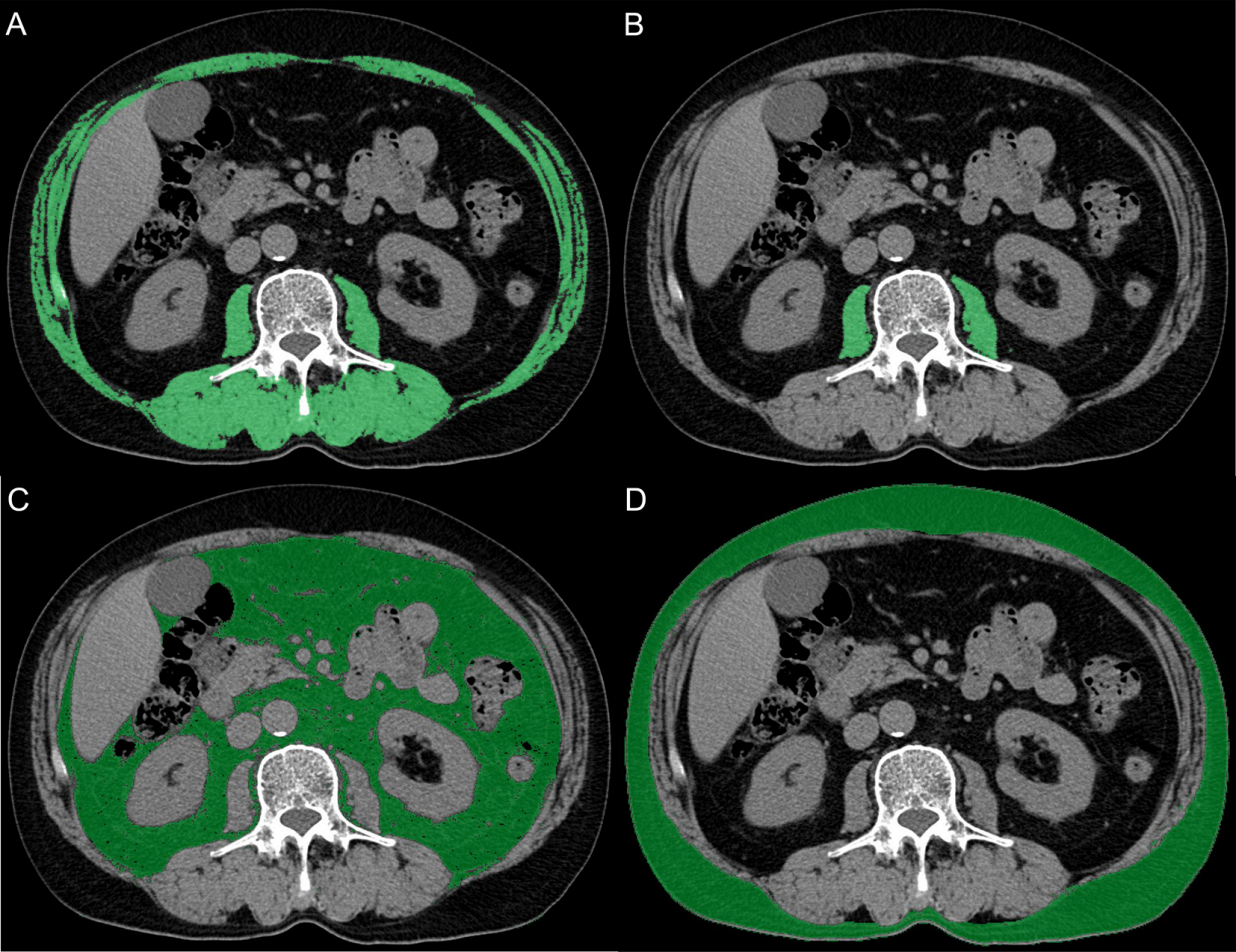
Computed tomography images of the third lumbar vertebra for each area. (a) Green highlights indicate the skeletal muscle area for the skeletal muscle index. (b) Green highlights indicate the psoas muscle area for the psoas muscle index. (c) Green highlights indicate the visceral adipose tissue area for the visceral adipose index. (d) Green highlights indicate the subcutaneous adipose tissue area for the subcutaneous adipose index.
